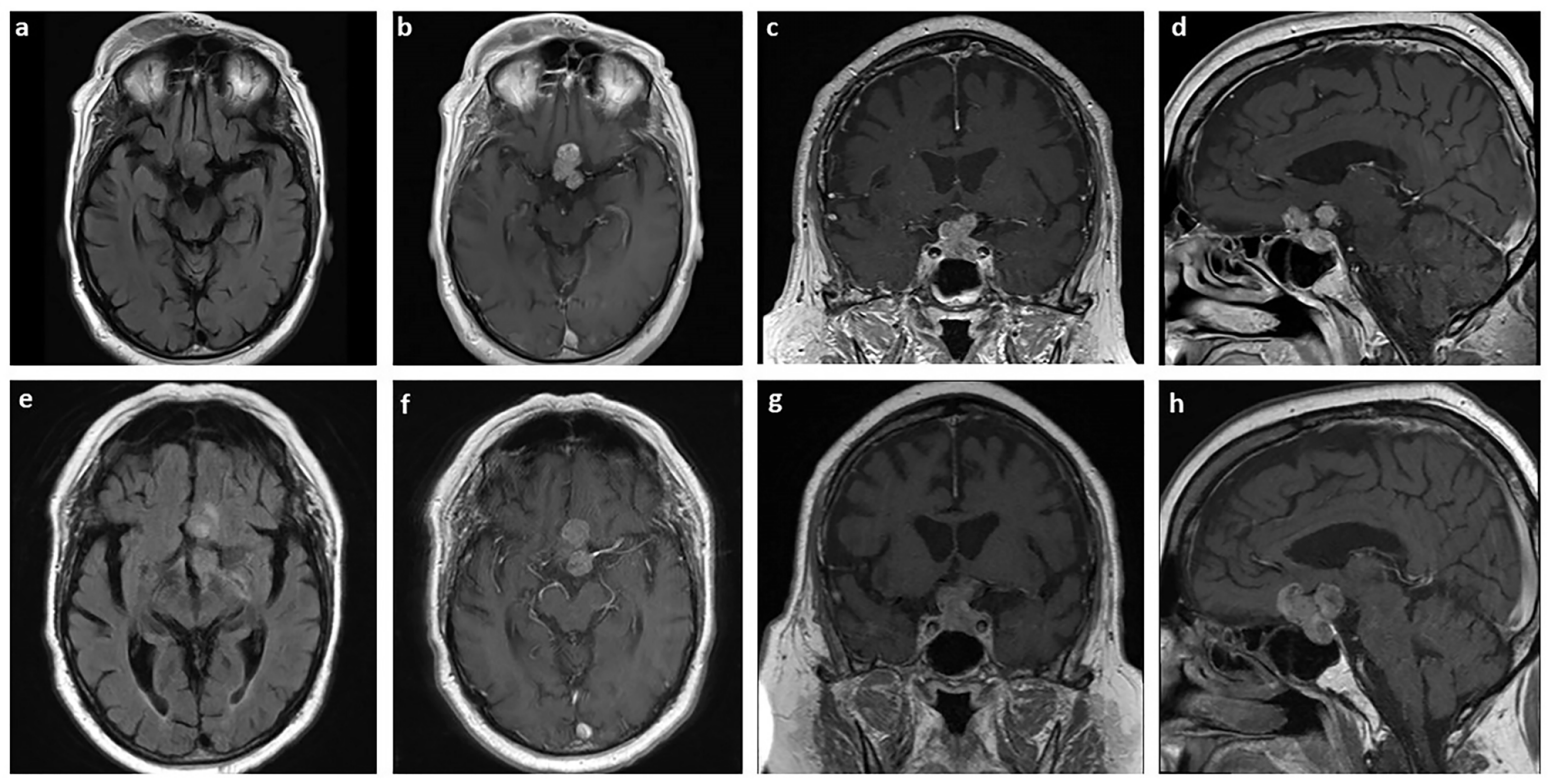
MRI of the pituitary sarcoma arising from a pituitary adenoma. Axial T2 FLAIR (A) and axial (B), coronal (C), and sagittal (D) T1-weighted with contrast MRI of tumor measuring 2 x 2.7 x 2 cm^3^ at the time of diagnosis. The same sections are shown for the tumor three years later, prior to surgical resection, measuring 3.3 x 3.0 x 2.6 cm^3^
